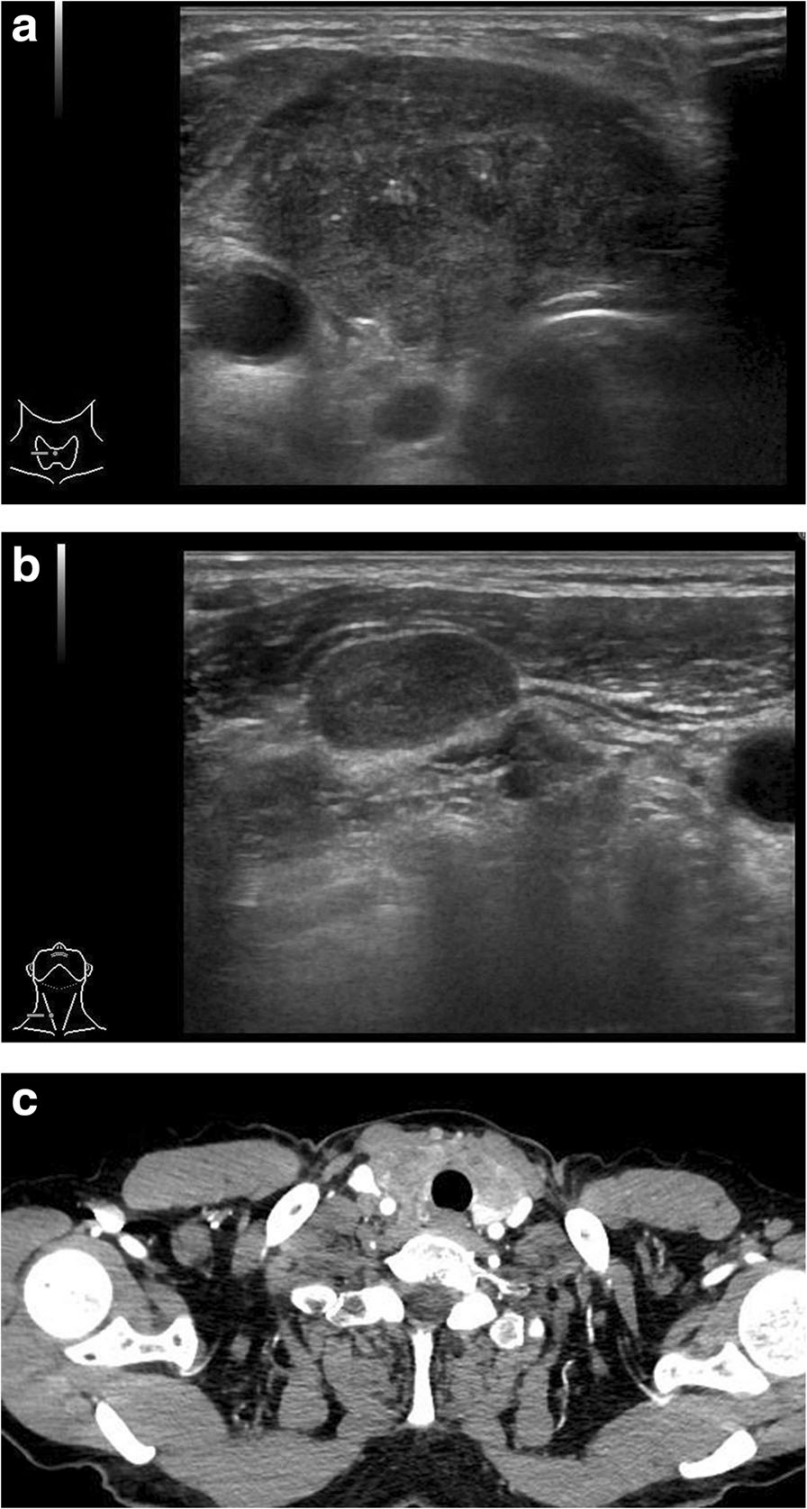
Ultrasound and CT scan findings. a Ultrasonography shows a hypoechoic mass in the thyroid. b Swollen bilateral cervical lymph nodes. c Low-density mass is spread in the thyroid with no evidence of invasion of the trachea
